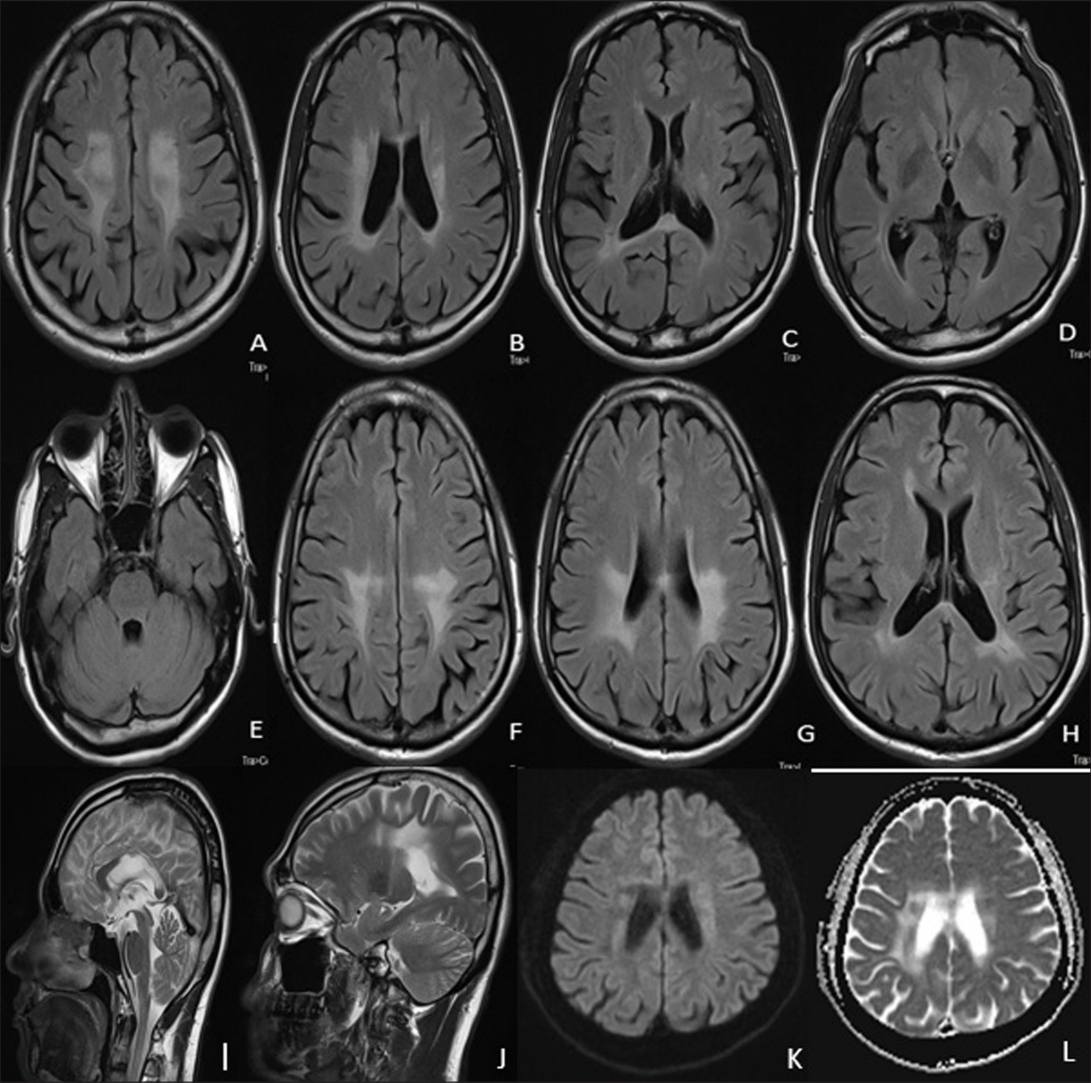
1A–1E: T2 FLAIR MR image showing diffuse bilateral white matter hyperintensities involving bilateral centrum semiovale, periventricular regions, splenium and forceps major, sparing frontal white and deep grey matter, brainstem and cerebellum. 1F–1H: T2 FLAIR images in patient’s brother with similar changes, albeit to a lesser extent. 1I–1J: T2 sagittal MR image shows corpus callosal body and splenial involvement along with parieto-occipital subcortical involvement. 1K–1L: Diffusion weighted imaging shows faint DW restriction in periventricular area with shine through on ADC (apparent diffusion coefficient) map.
